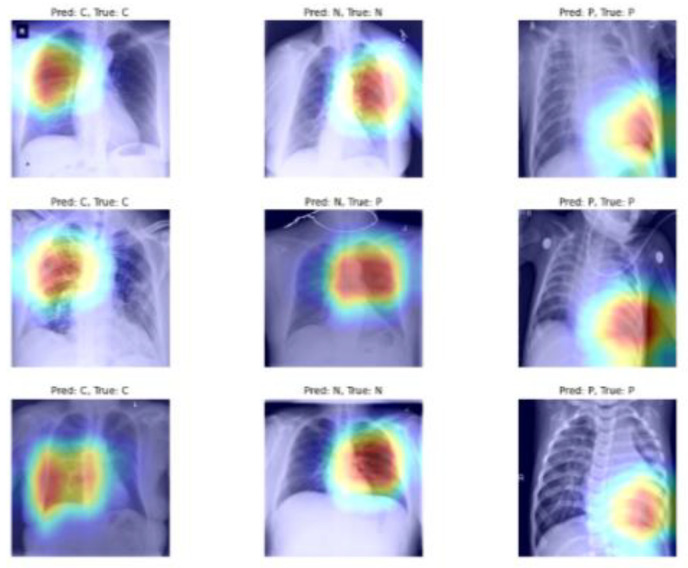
Raw chest X-ray image corresponding to Figure 9 and Grad-CAM visualization generated using the following model: Xception model + coordinated attention module + LSTM layer + new classification head.
